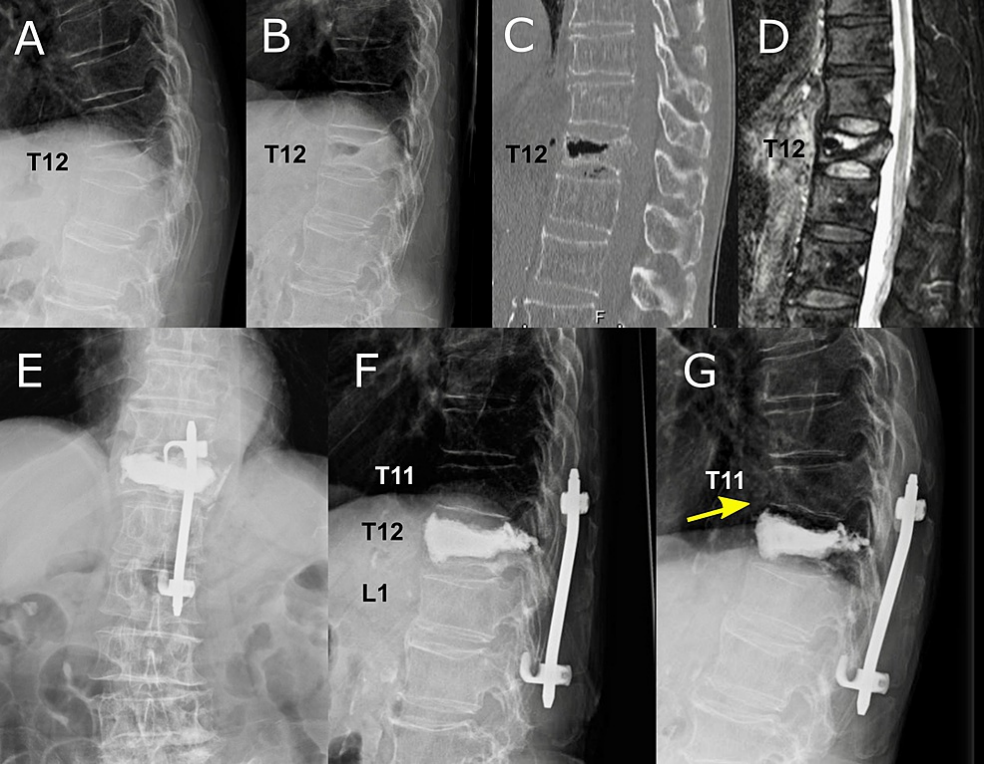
Another case of OVF treated by BKP with minimally invasive posterior hook stabilization (Case 4 in Table 1) An 86-year-old man showing severe instability of T12 OVF (A: sitting position, B: supine position). Computed tomography showed a vacuum cleft in the fractured vertebra (C). Magnetic resonance imaging showed a very high signal intensity in the T12 vertebra (D). BKP augmented with minimal invasive posterior hook stabilization was performed (E, F). He suffered an adjacent fracture of T11 (G: yellow arrow) but it healed successfully after conservative treatment. BKP, balloon kyphoplasty; OVF: osteoporotic vertebral fracture
